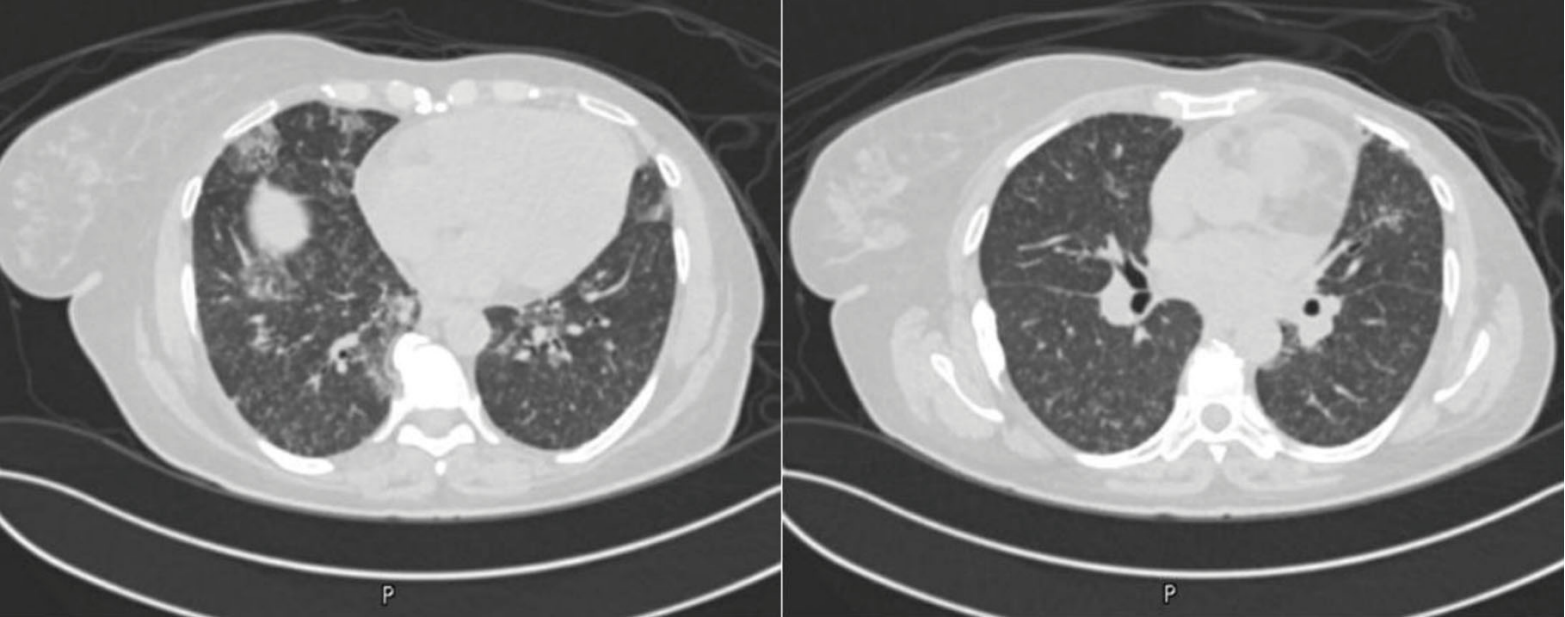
Chest CT scan showed diffuse numerous tiny nodules in both lung fields with areas of patchy GGOs and intralobular thickening more pronounced in both lower lobes.
